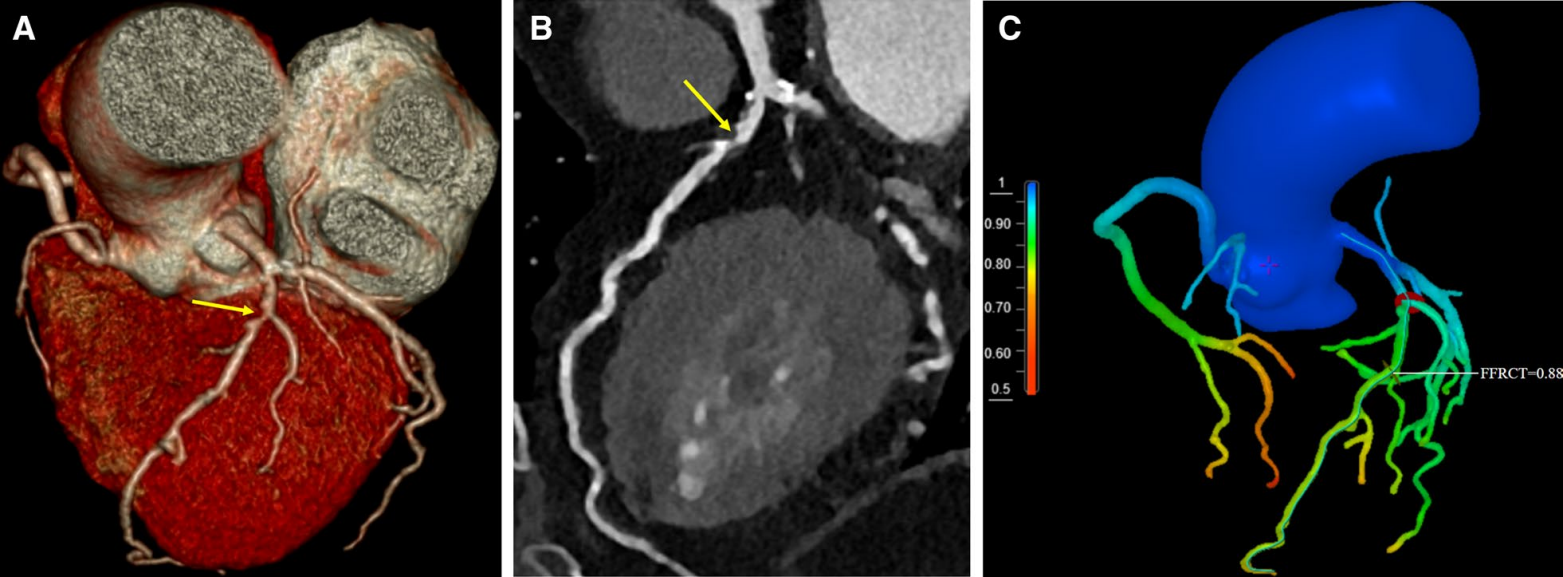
Representative case of patient without MACE. A 78-year-oid female with unstable angina. CCTA showed mixed plaque with moderate stenosis at proximal LAD (yellow arrow in A, B). This lesion was revealed to be hemodynamically insignificant with FFRCT value of 0.88 (C). The patient was treated with medical therapy and was followed up for 24 months without MACE. CCTA, coronary computed tomography angiography; FFRCT, fractional flow reserve derived from computed tomography; MACE, major adverse cardiac events; LAD, left anterior descending
